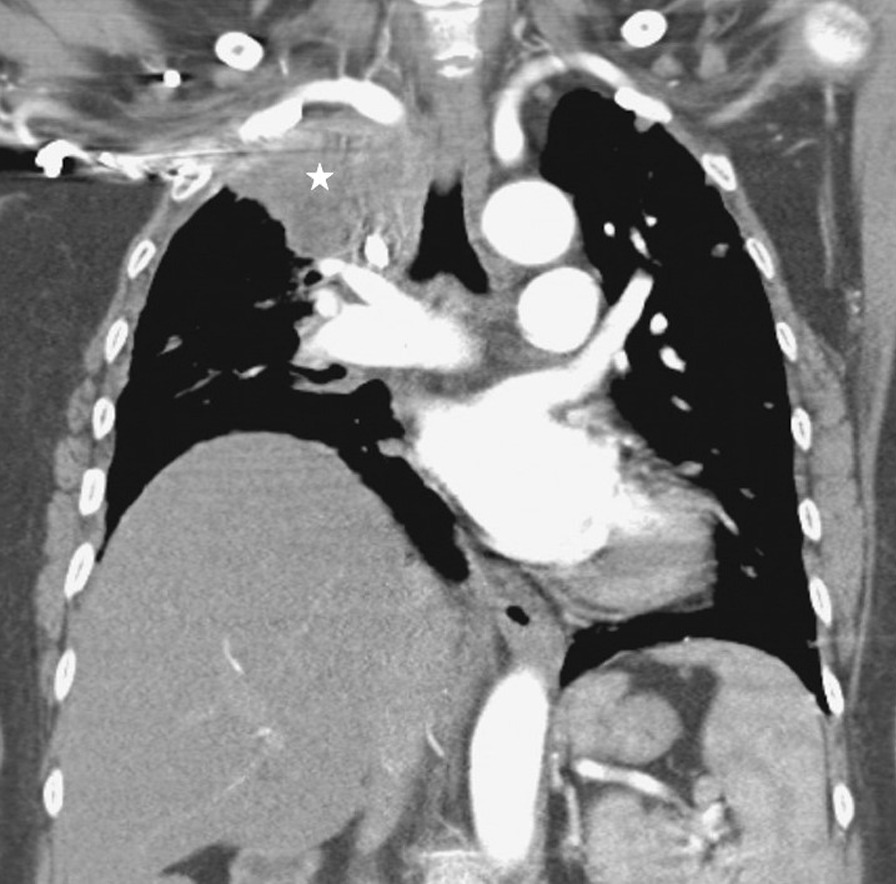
Right hemidiaphragm paralysis due to phrenic nerve invasion by apical pulmonary metastasis from colorectal carcinoma. Coronal CT image shows right apical mass (star) and right hemidiaphragm elevation. Case coutesy of Assoc Prof Frank Gaillard, Radiopaedia.org, rID: 8543
